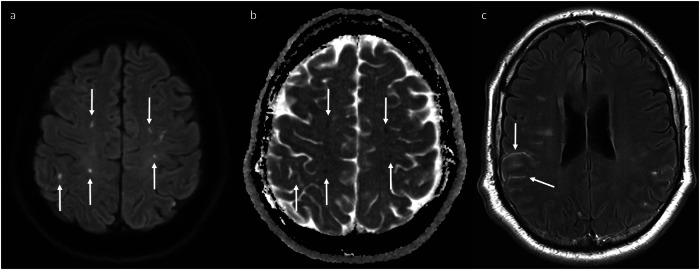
This patient developed evidence of irAE vasculitis while on maintenance nivolumab for unresectable stage III melanoma. Diffusion-weighting imaging (a) and apparent diffusion coefficient map (b) show foci in both cerebral hemispheres exhibiting high and low signal (arrows), respectively, consistent with recent infarcts in multiple vascular territories. The patient also had abnormal sulcal leptomeningeal enhancement on the post-contrast FLAIR sequence (c, arrows). The leptomeningeal enhancement subsequently resolved after treatment with intravenous methylprednisolone and cyclophosphamide, confirming that it was related to the irAE-vasculitis rather than leptomeningeal metastatic disease
